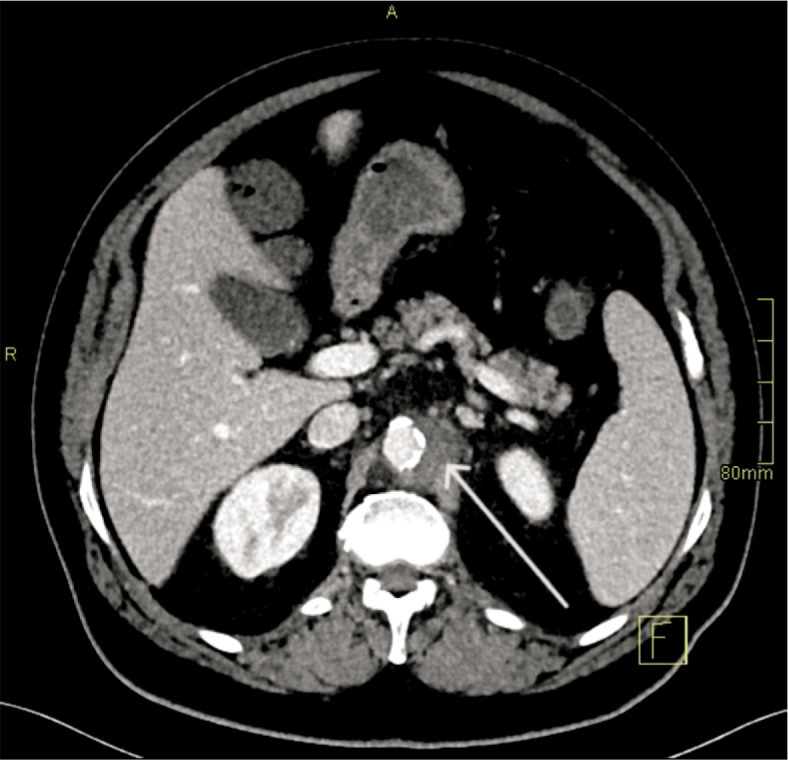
Aortitis (Day 1). Axial CT angiography (CTA) demonstrates circumferential wall thickening and periaortic fat stranding in the paravisceral aorta, without a focal outpouching (arrow).
